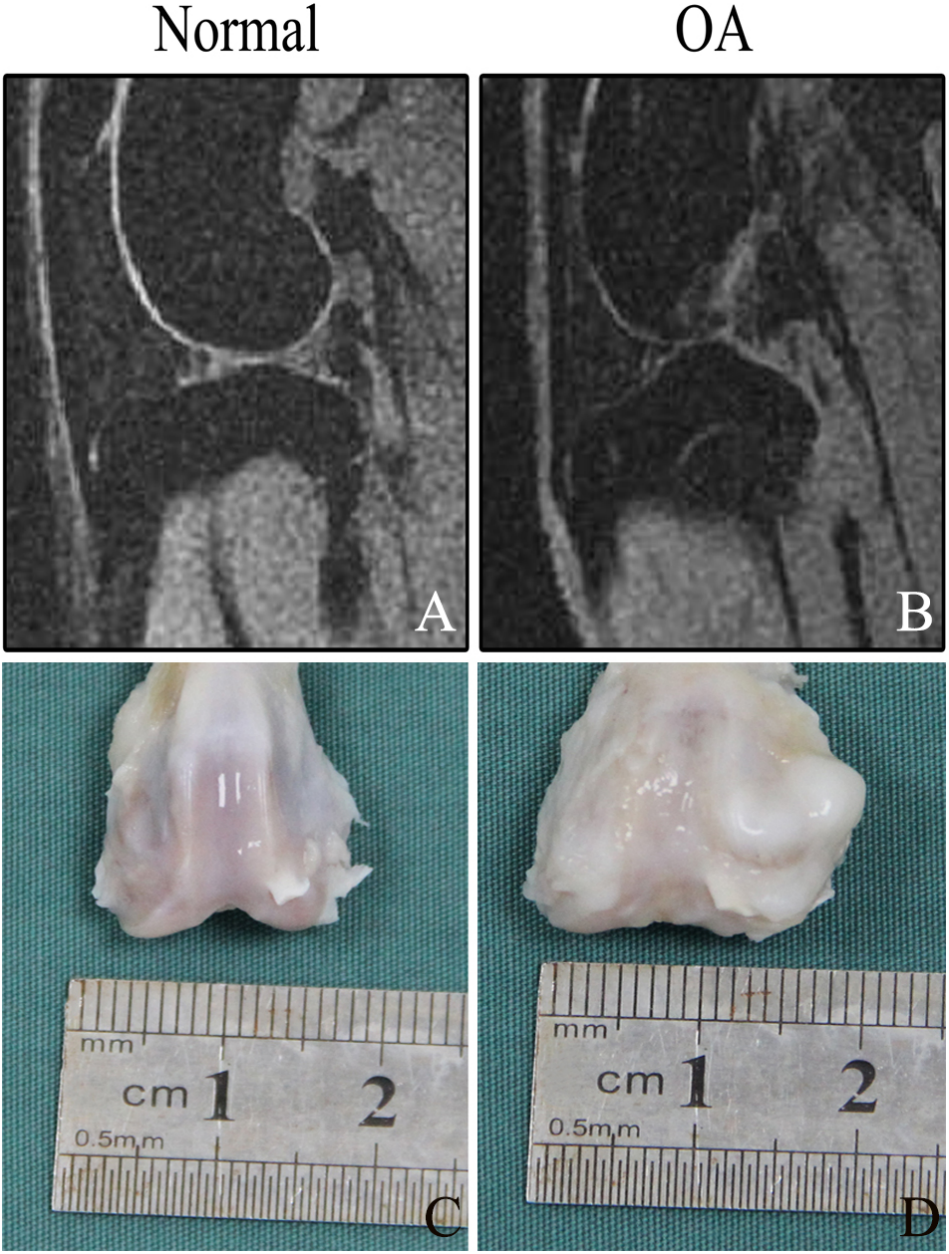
Evaluation of osteoarthritis model. (A) The cartilage continuity was intact under magnetic resonance imaging, and no obvious damage was seen. (B) The continuous destruction of the cartilage and the thinning of the cartilage layer were observed under magnetic resonance imaging. (C) The cartilage surface was smooth with no obvious damage. (D) The cartilage surface shows erosion and the integrity of the surface cartilage was disrupted.
